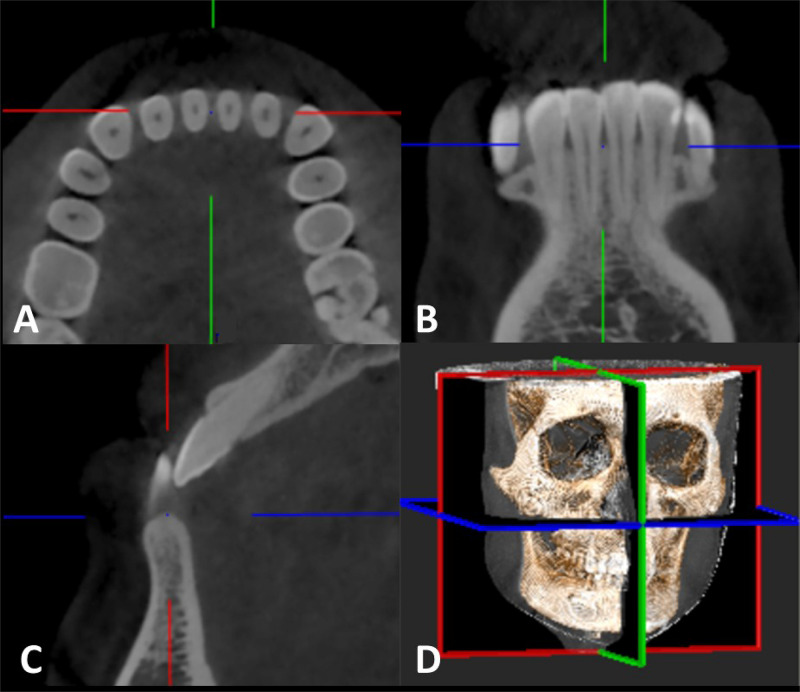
CBCT scanning and 3D reconstruction: (A) horizontal view; (B) coronal view; (C) sagittal view; and (D) three-dimensional reconstruction.
